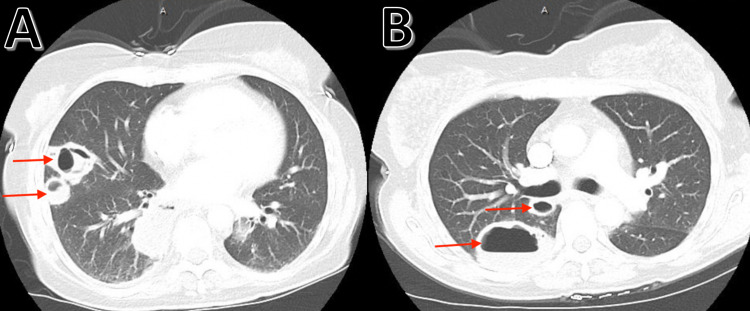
Computed tomography angiography of the chest showing non-cavitary lesions (A: lower red arrow) and cavitary lesions (A: upper red arrow and B: both red arrows), with the largest cavitary lesion being seen in the superior segment of the right lower lobe (B: lower red arrow)
